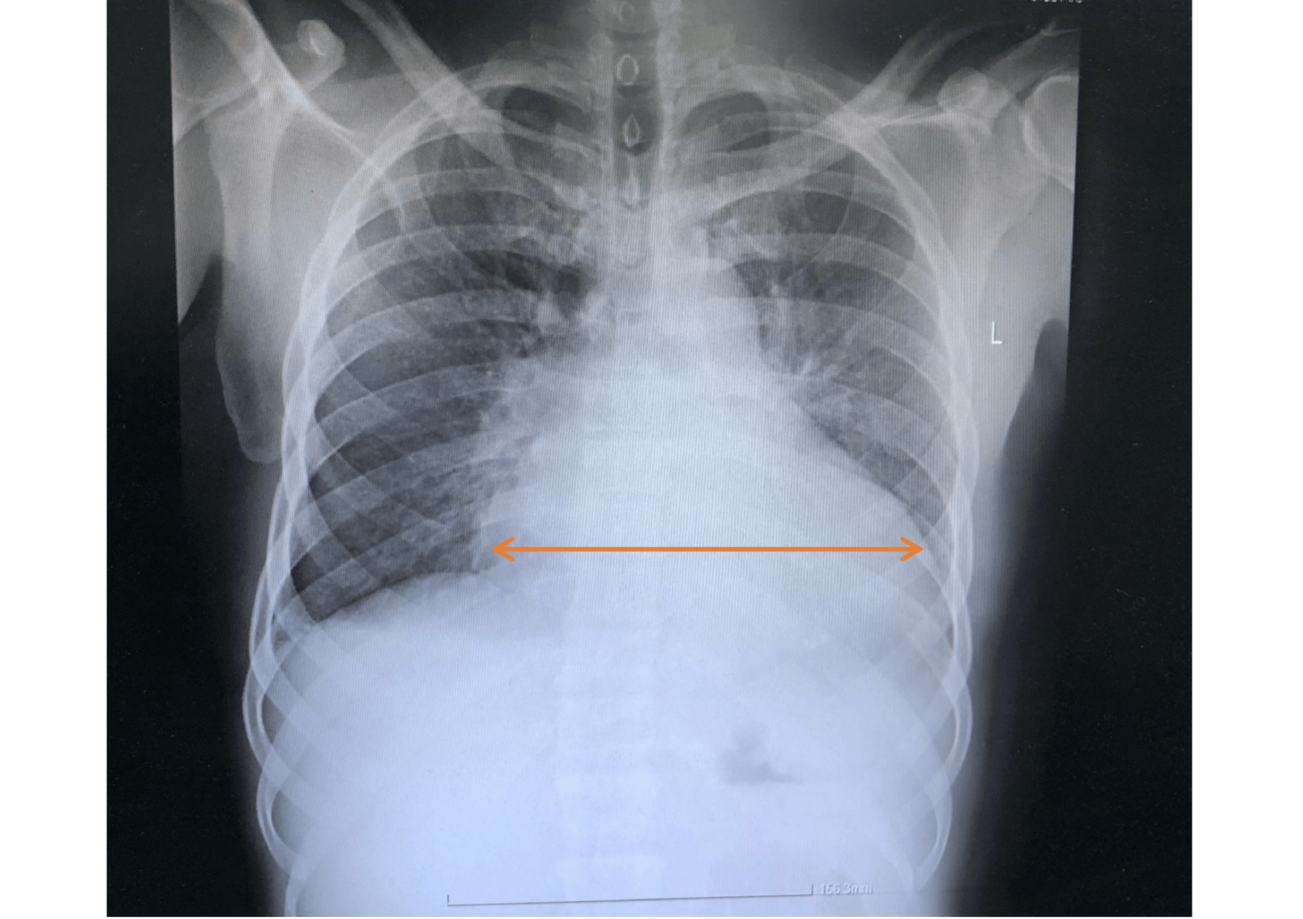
Erect chest X-ray showing cardiomegaly with a cardiothoracic ratio of 0.75 (arrow)
